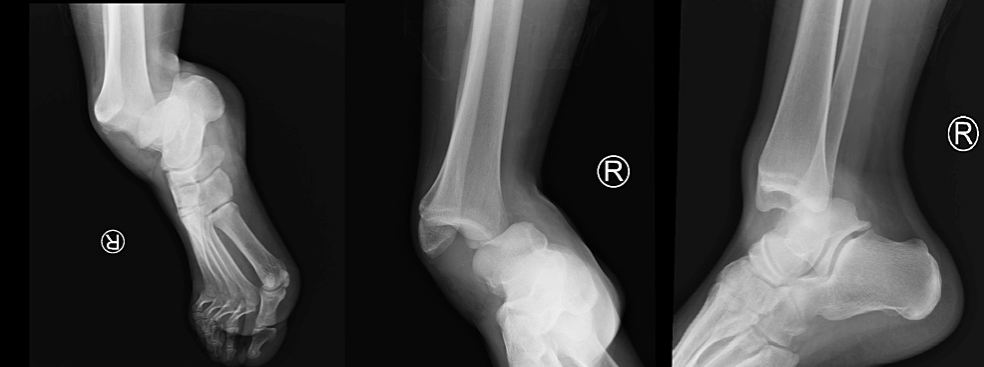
Posteromedial pure ankle dislocation. Anteroposterior and lateral radiographs.
